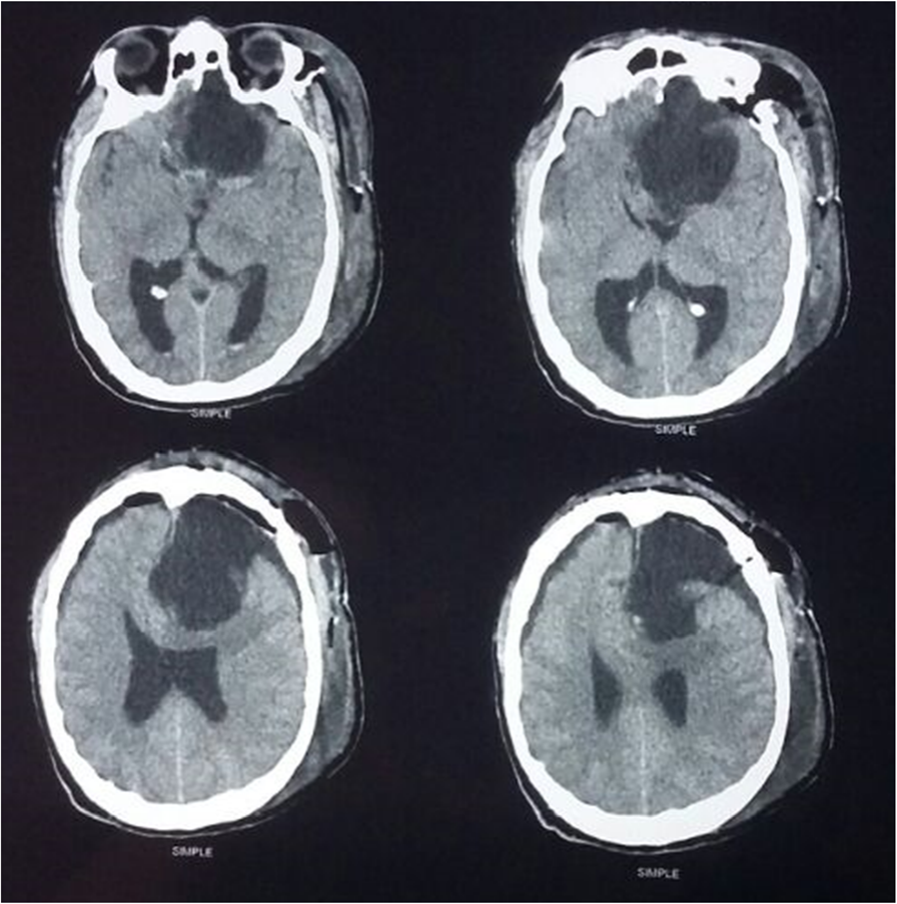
Postoperative non enhanced CT scan image showing axial cut after tumor resection with cavity occupied by cerebrospinal fluid (performed two months after the patient was discharged from the Psychiatric Hospital)
